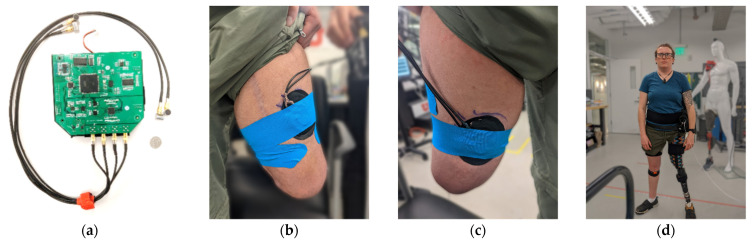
Sensor placement: (a) A-mode ultrasound system, with dime for scale. (b) Anterior US transducers placement. (c) Posterior US transducers placement. (d) Subject equipped with US system and IMU sensors.
